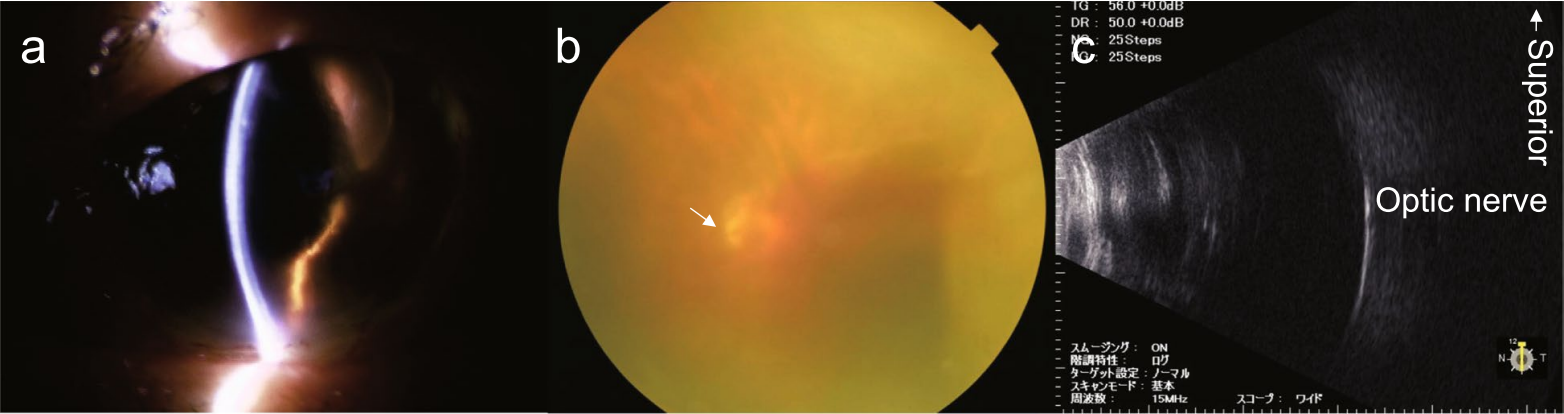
Postoperative findings at 7 days after the second surgery. No bleeding is observed in the anterior chamber during a slit-lamp examination (a). The optic disc and coagulation site are observed in the posterior fundus (b). Ocular ultrasound examination does not show any hyperechoic images suggestive of vitreous hemorrhage (c)
